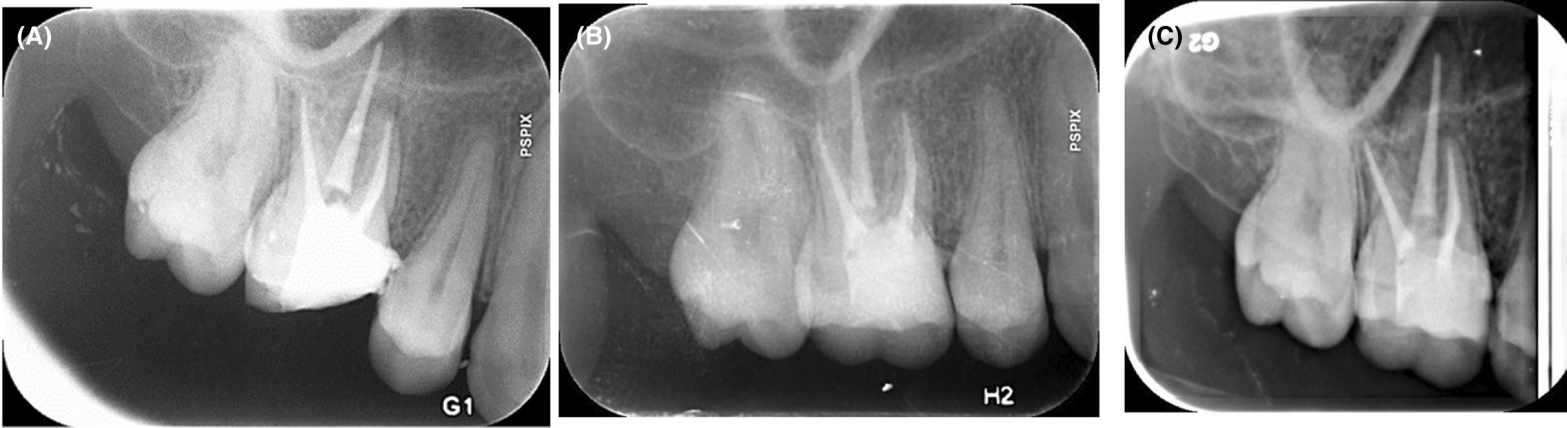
Post‐op periapical radiograph (A), periapical radiograph of 4‐month recall (B), and 12‐month recall (C).
